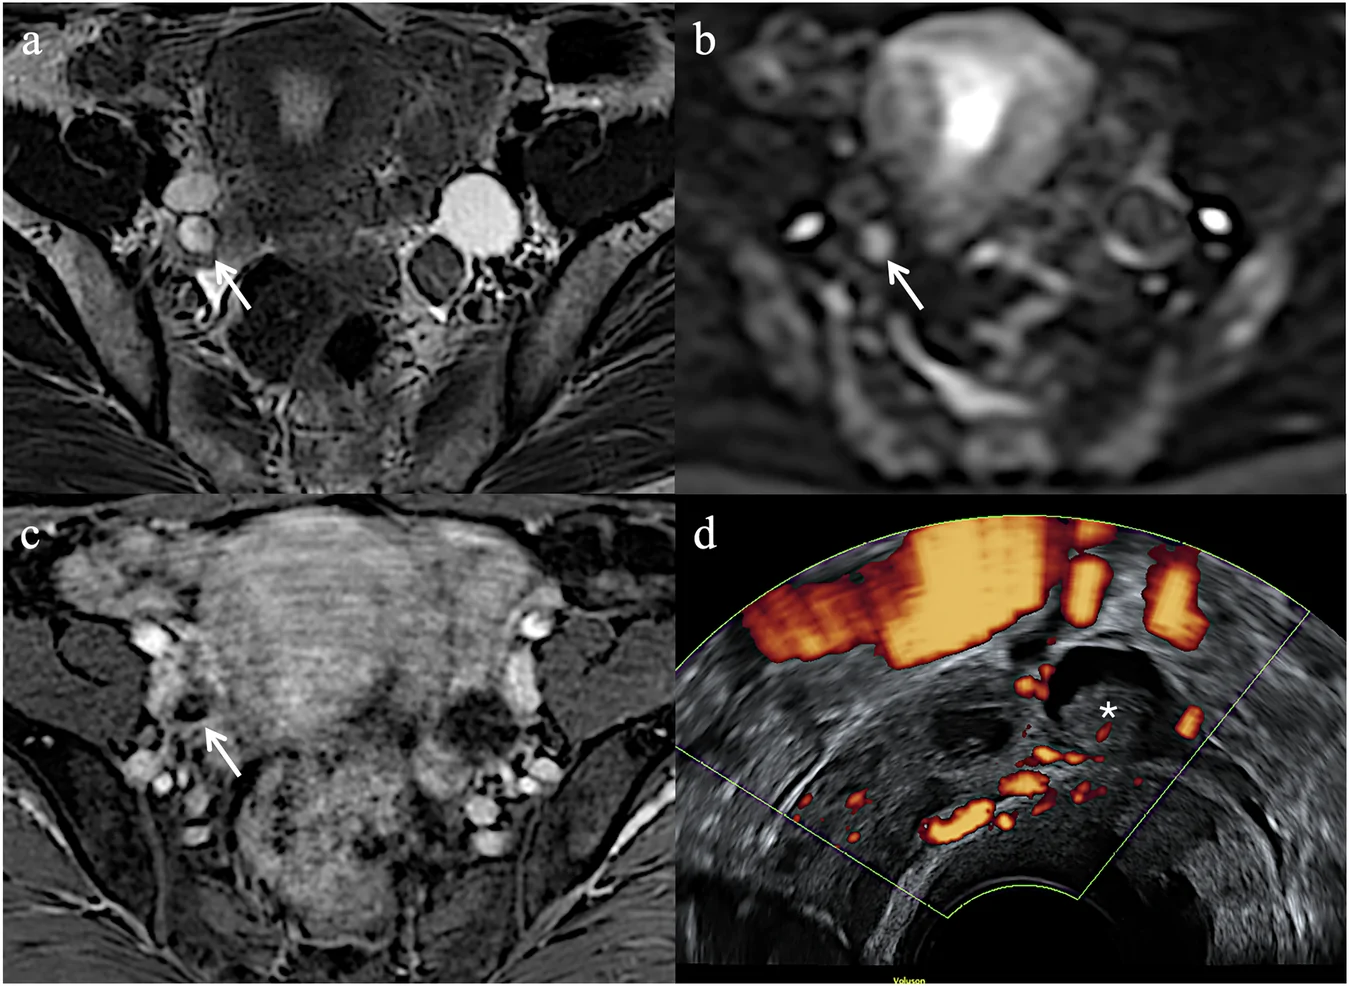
Impact of second-look ultrasonography. a Axial T2-weighted sequence; b axial DWI; c axial postcontrast fat-saturated T1-weighted sequence; d second-look ultrasonography. Case of a 25-year-old patient referred for cystic adnexal lesion characterization. MRI shows a right cystic locule with intermediate T2W signal and high diffusion signal (white arrow). The postcontrast sequence shows equivocal enhancement within the locule. Second-look ultrasonography confirmed a papillary projection (*). The lesion was classified as O-RADS MRI score 4, and a borderline serous tumor was suspected. Surgical management confirmed the diagnosis. This case highlights the potential value of second-look ultrasonography in detecting small solid tissue
